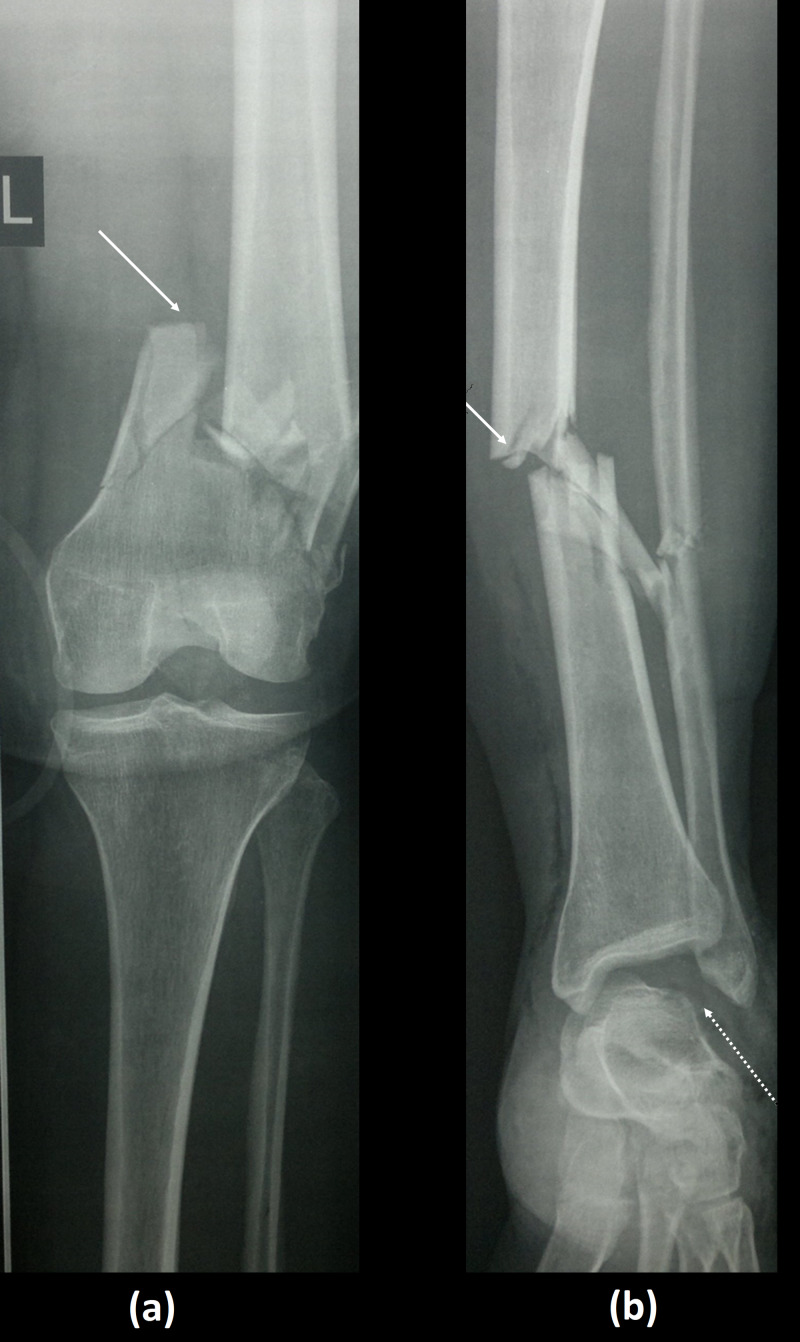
(a) AP radiograph of the left knee showing left extraarticular distal femur fracture. (b) AP radiograph of the left leg showing tibial shaft fracture (solid white arrow), and a complete absence of the talar body (dotted arrow). AP, anteroposterior
